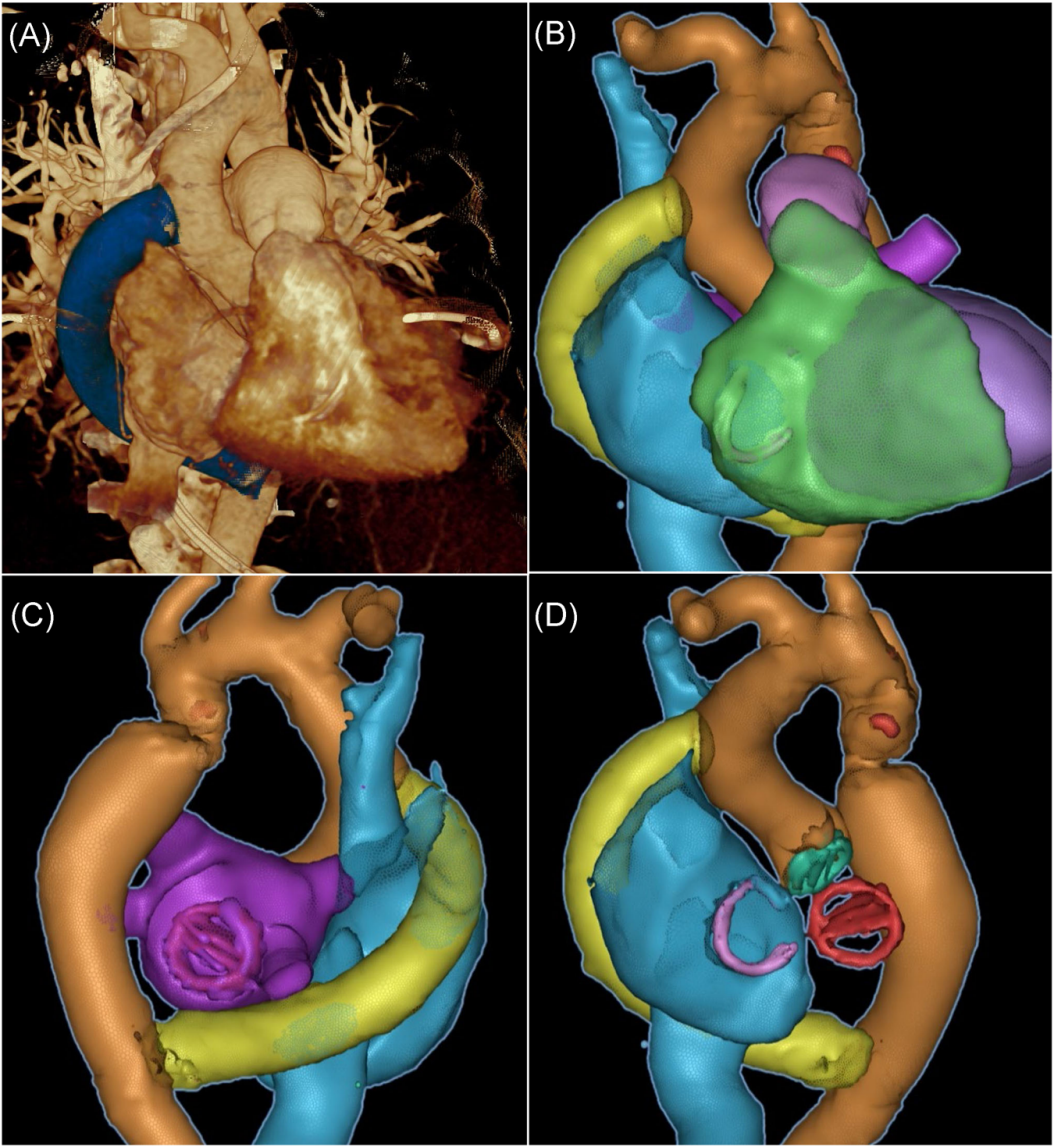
Postoperative computed tomography (CT) of ascending‐to‐descending bypass routed behind the inferior vena cava. (A) Three‐dimensional segmentation highlighting the bypass routing. (B) CT‐based reconstruction of ascending‐to‐descending bypass (yellow), cardiac structures and implants. (C) Aortic (green) and mitral valve (red) prostheses and tricuspid ring (purple). (D) Posterior view of ascending‐to‐descending bypass showing calcifications in the isthmus area (red)
